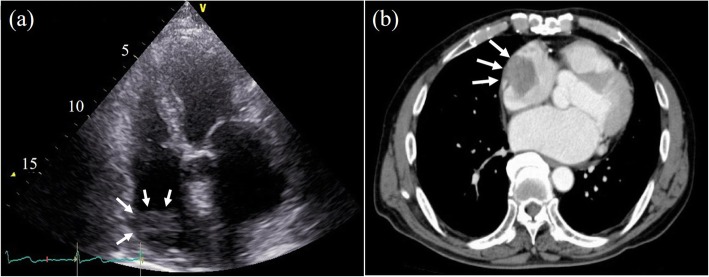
a A 45 × 27 mm mass (white arrows) was observed as an area of low density near the right atrial free wall on preoperative contrast-enhanced computed tomography. b A round floating mass (white arrows) with a relative low echogenicity was recognized in the right atrium in the preoperative four-chamber view of transthoracic echocardiography
